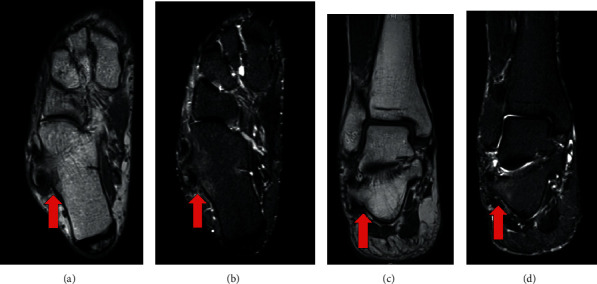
Right foot MRI: T1-weighted axial image (a), STIR axial image (b), T1-weighted coronal image (c), and STIR coronal image (d). Low-intensity lesion on T1-weighted image and high-intensity lesion on STIR image were detected around hypertrophic peroneal tubercle, and bone marrow lesions were suggested (red arrow).
